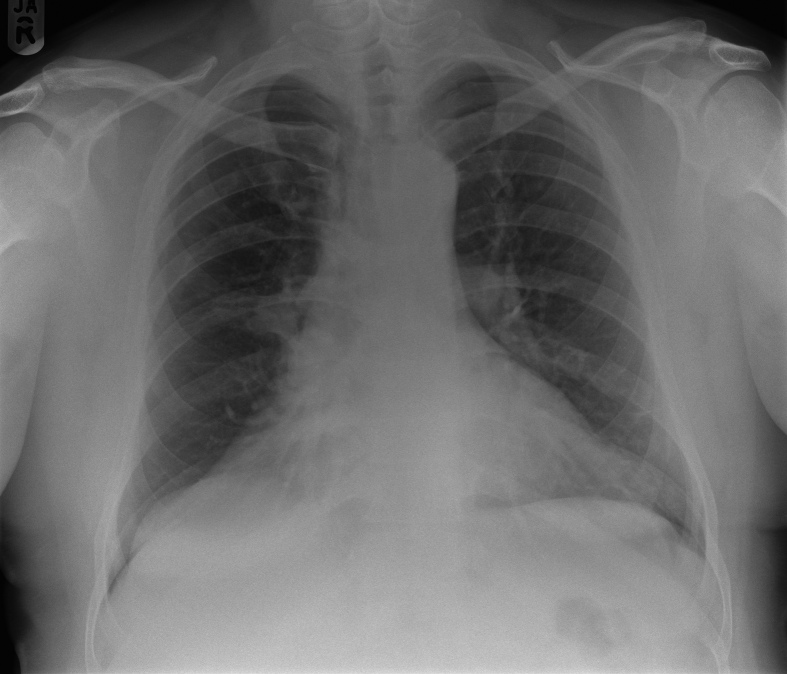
Pulmonary angiogram chest radiograph at presentation demonstrates right lung volume loss and a mid-zone opacity effacing the right cardiac border.
